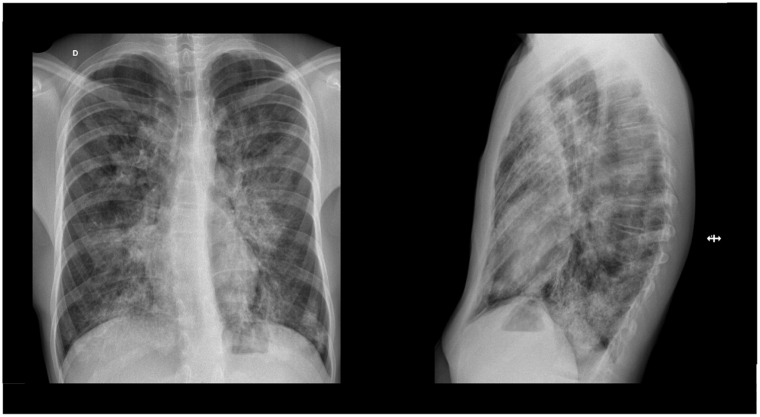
Chest radiograph showing marked bilateral interstitial infiltrate.
